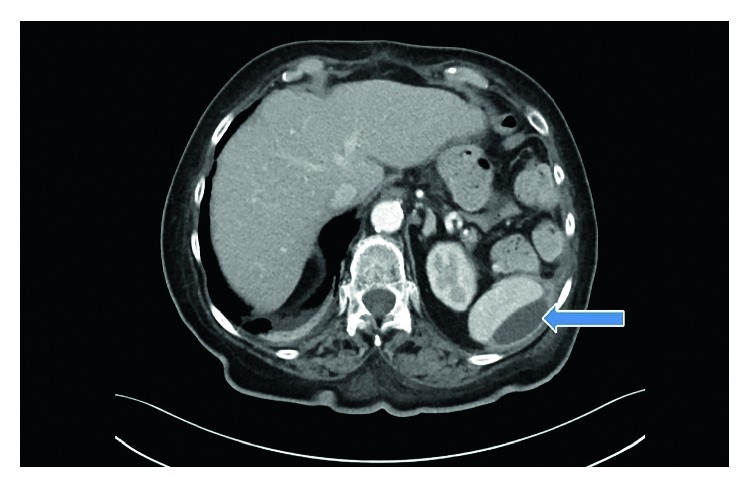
CT scan showing subcapsular fluid formation of the spleen as manifestation of septic herds in endocarditis.
